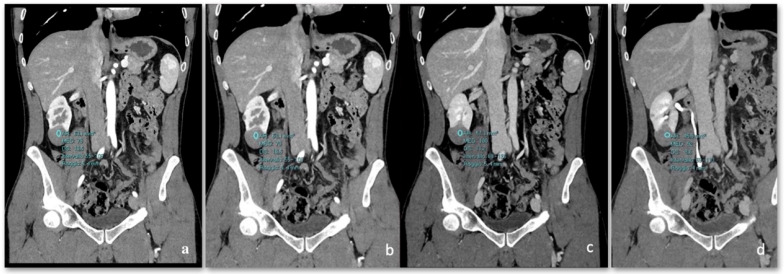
(a) Coronal non-enhanced CT shows a slightly hyperdense round lesion at the lower pole of the right kidney (36 HU). (b) In the arterial phase, the lesion shows a minimal enhancement of 79 HU. (c) In the nephrogenic phase, minimal and delayed enhancement is observed, reaching 100 HU. (d) In the delayed phase, the lesion shows no significant washout at 92 HU.
